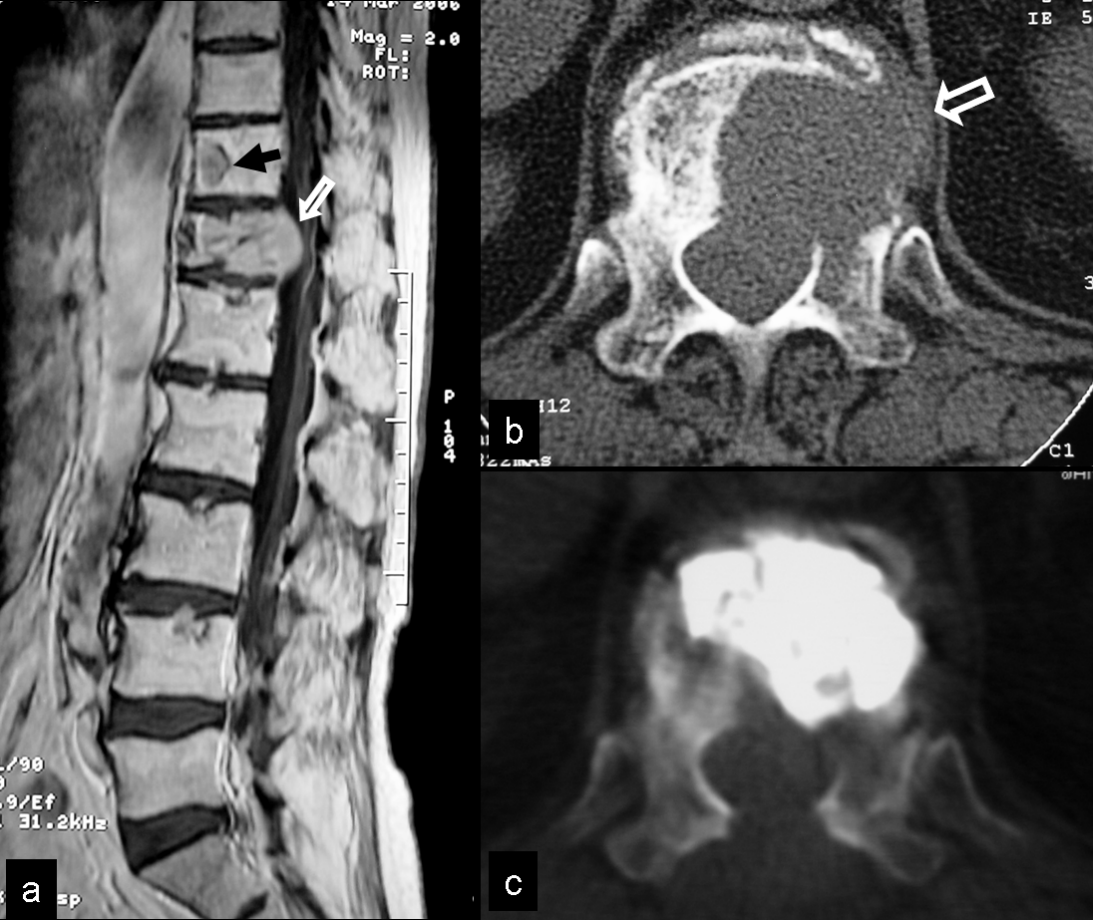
Malignant fracture of the T12 vertebral body, secondary to hepatocellular carcinoma. α) The sagittal contrast enhanced T1-w TSE MR image shows the malignant fracture with posterior displacement (arrow). An additional secondary deposit is shown in the T11 vertebral body (black arrow). The axial CT images show the preoperative osteolysis (arrow in b) and the postoperative result (c).
